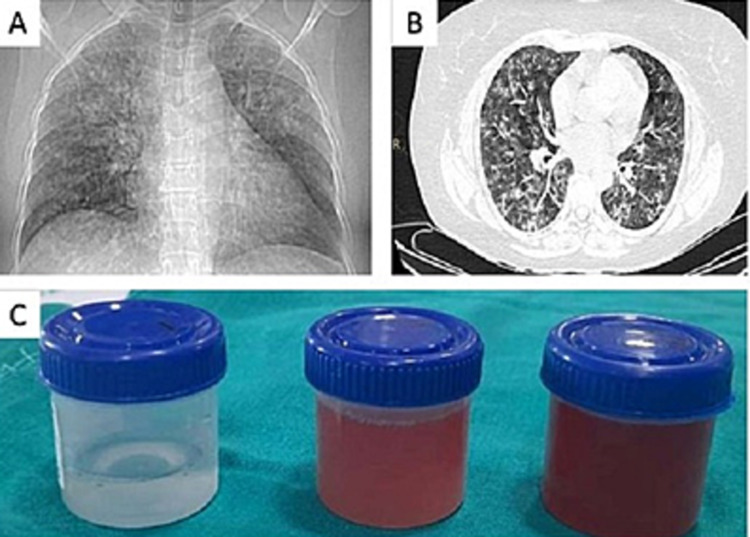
(A) Chest x-ray showing bilateral opacities. (B) Computed tomography (CT) chest showing diffuse ground glassing involving both lungs. (C) Broncho-alveolar lavage (BAL) showing progressive bloody return.
